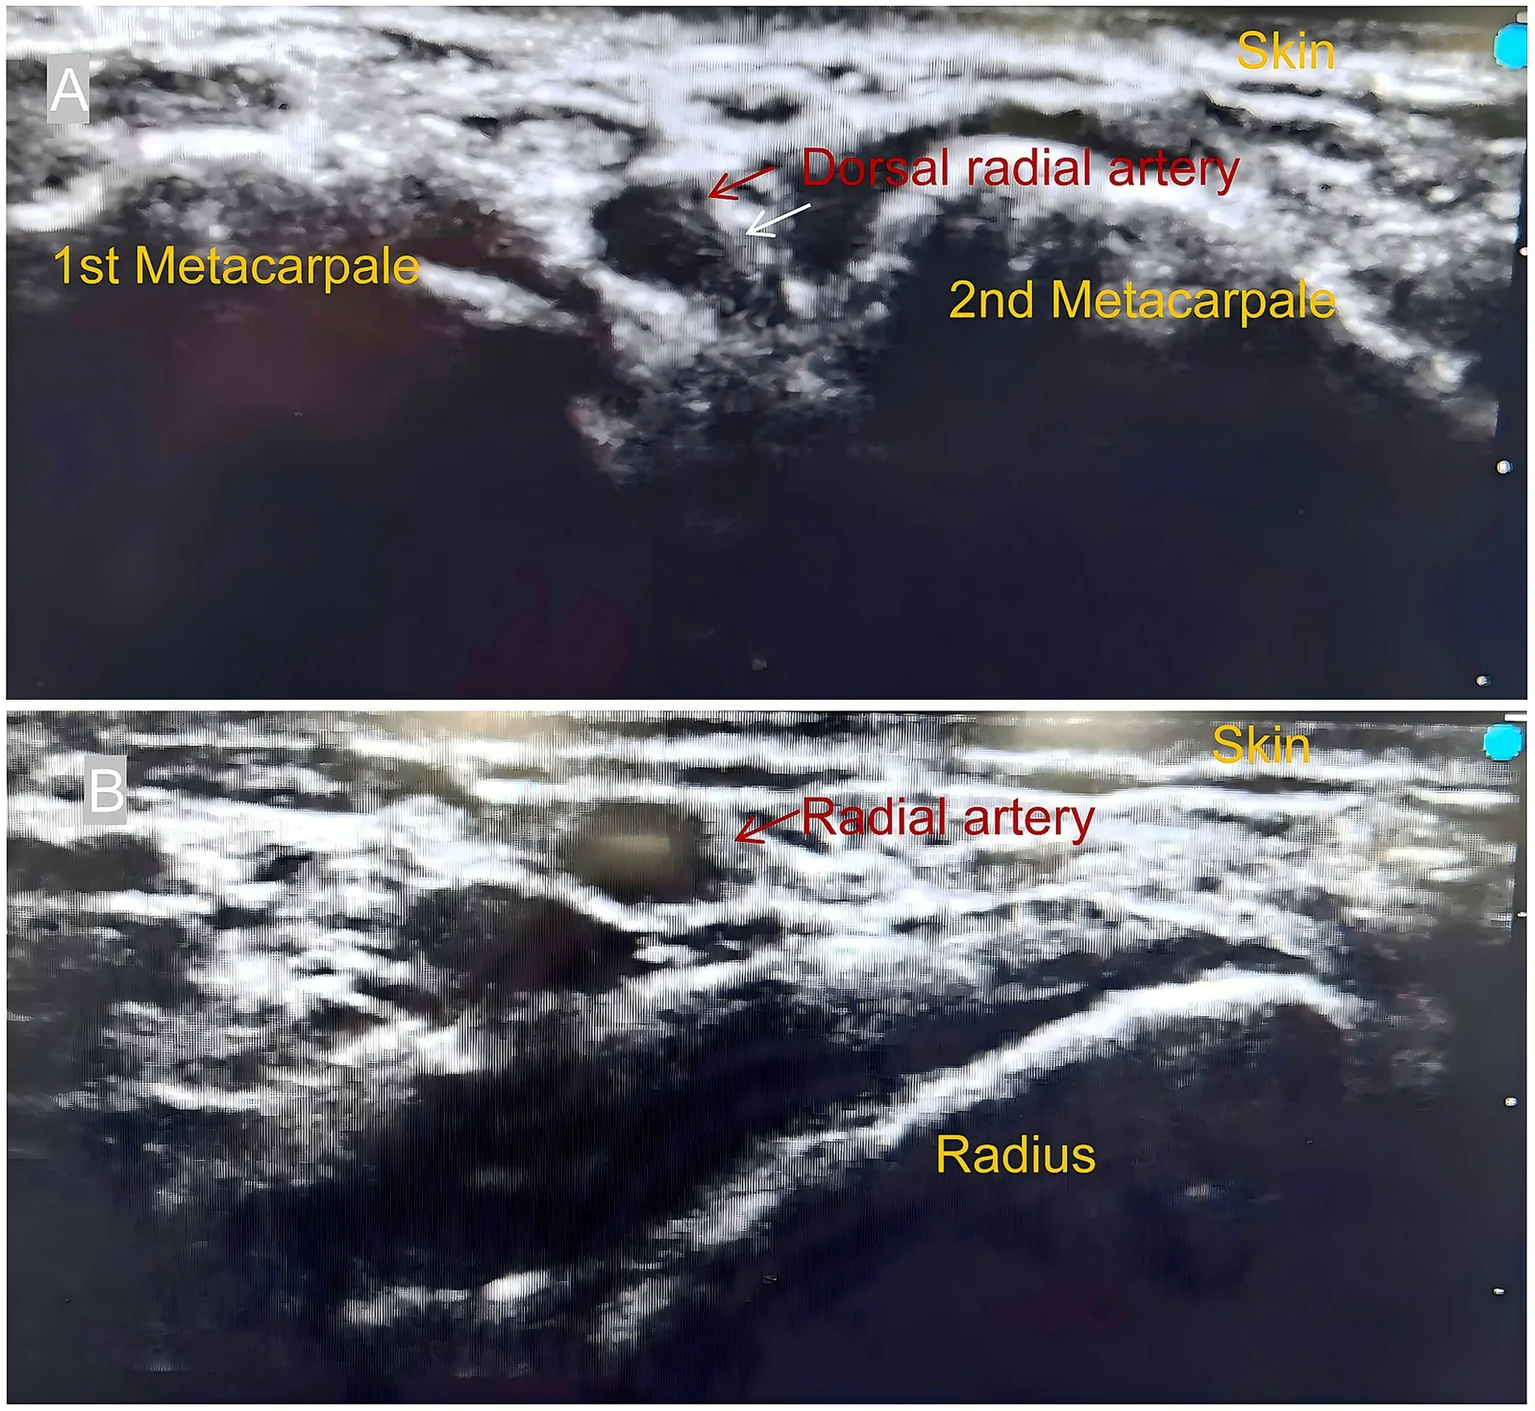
Comparison of ultrasound images of the distal radial artery in the Hegu region and the wrist radial artery. (A) The ultrasound images of the distal radial artery in the Hegu region; (B) The ultrasound images of the wrist radial artery.
